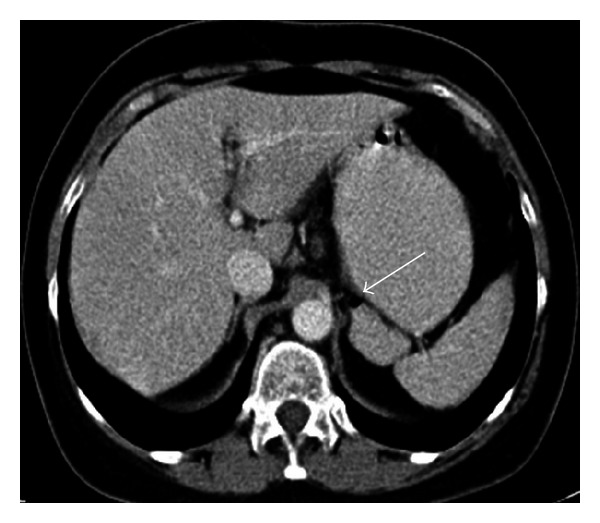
Axial contrast enhanced CT image with oral contrast medium reveals a hyperdense mass posterior to the stomach and medial to the spleen. The gas bubble anterior to the mass (arrow) helps gastric diverticulum in differentiating it from an accessory spleen.
